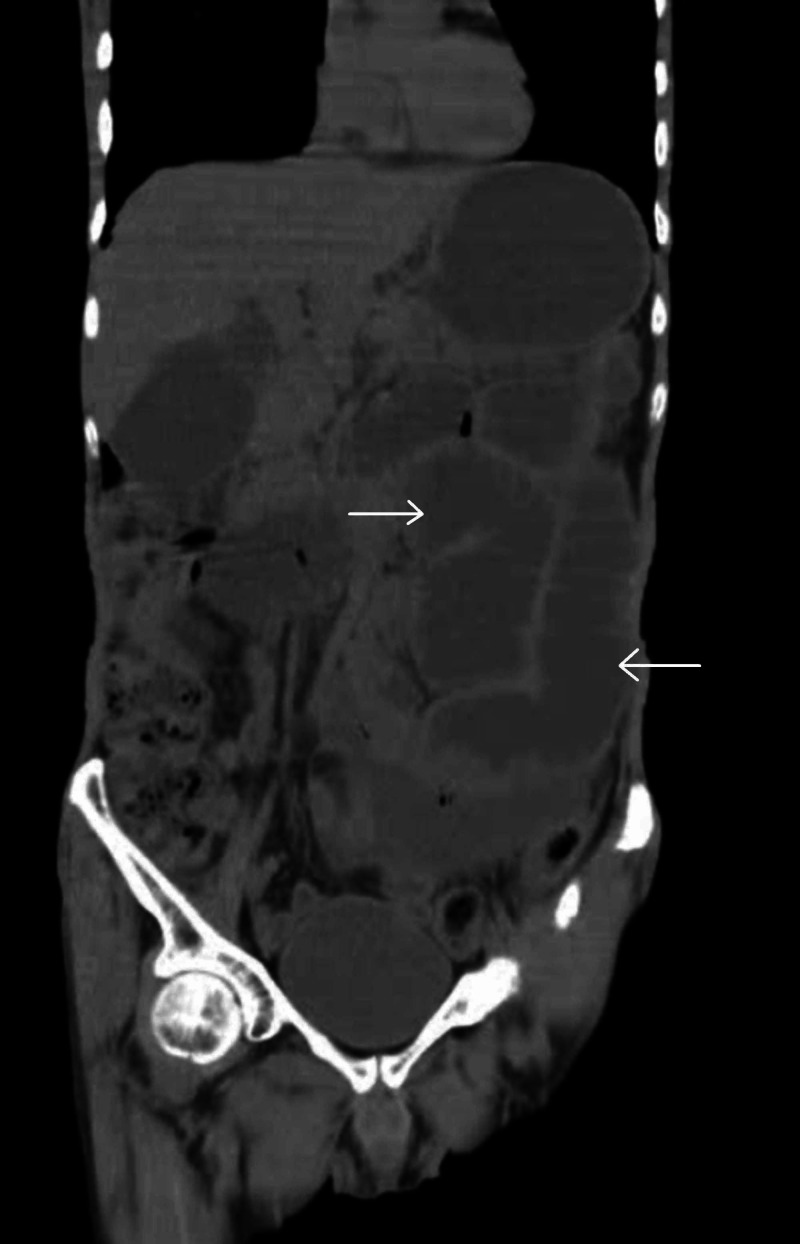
Preoperative coronal computed tomography (CT) scan of the abdomen showing dilated small bowel loops (arrows).
